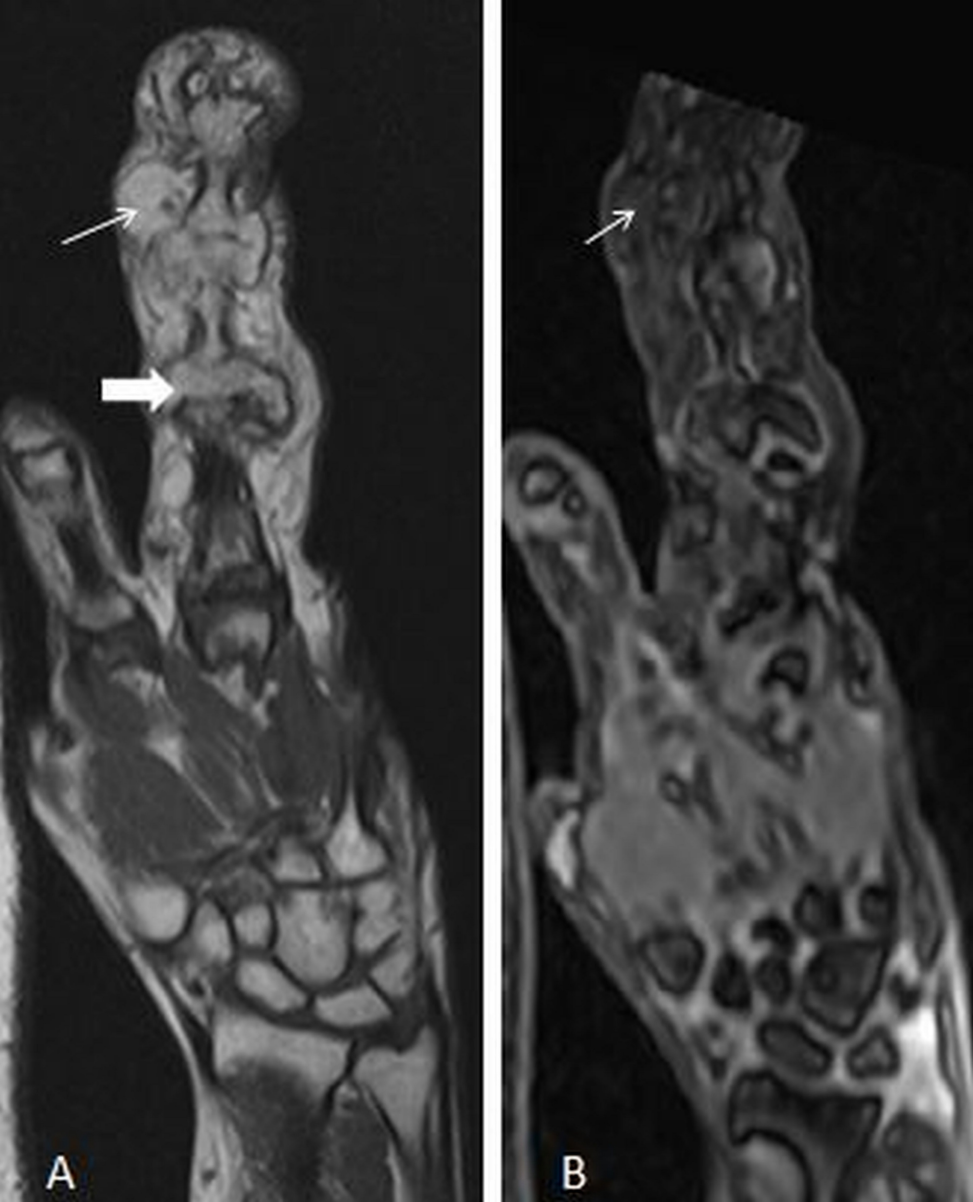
A) T1-weighted coronal image shows lipomatous hypertrophy of the third digit (thin arrow) with secondary osteoarthritic changes in the interphalangeal joints (thick arrow). B) T1 FS coronal image shows suppression of hypertrophied fat tissue in the third digit (thin arrow). Underlying marrow is not involved.
